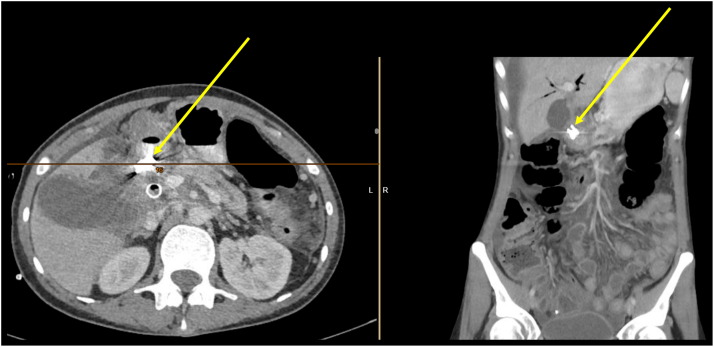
CT scan with oral contrast confirming sufficient closure of the duodenal wall. *Arrow* indicates the clips.
